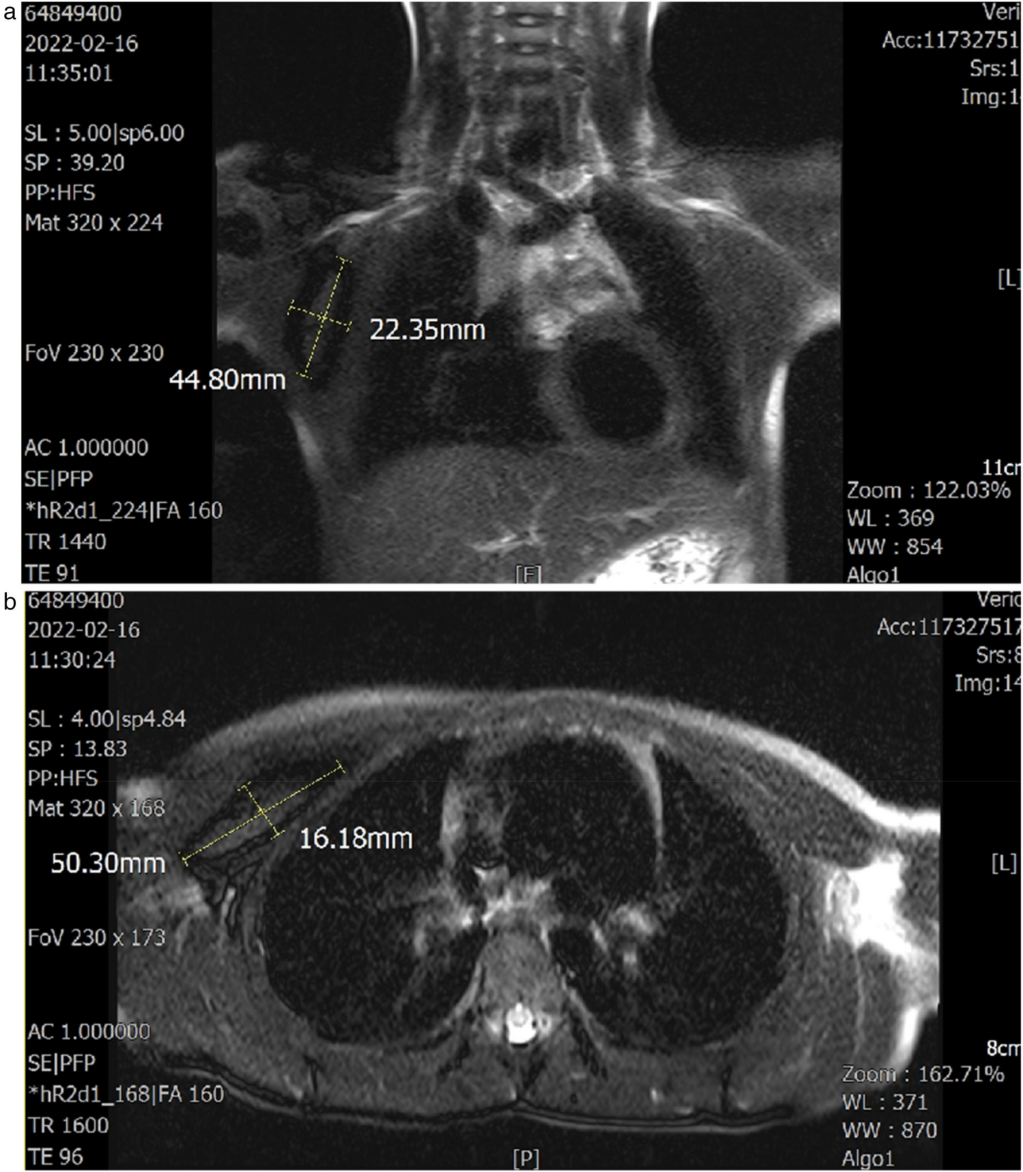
(a,b) Axial and coronal T1- and T2-weighted images demonstrate the lesion site and dimensions. The sagittal plane was unremarkable.
